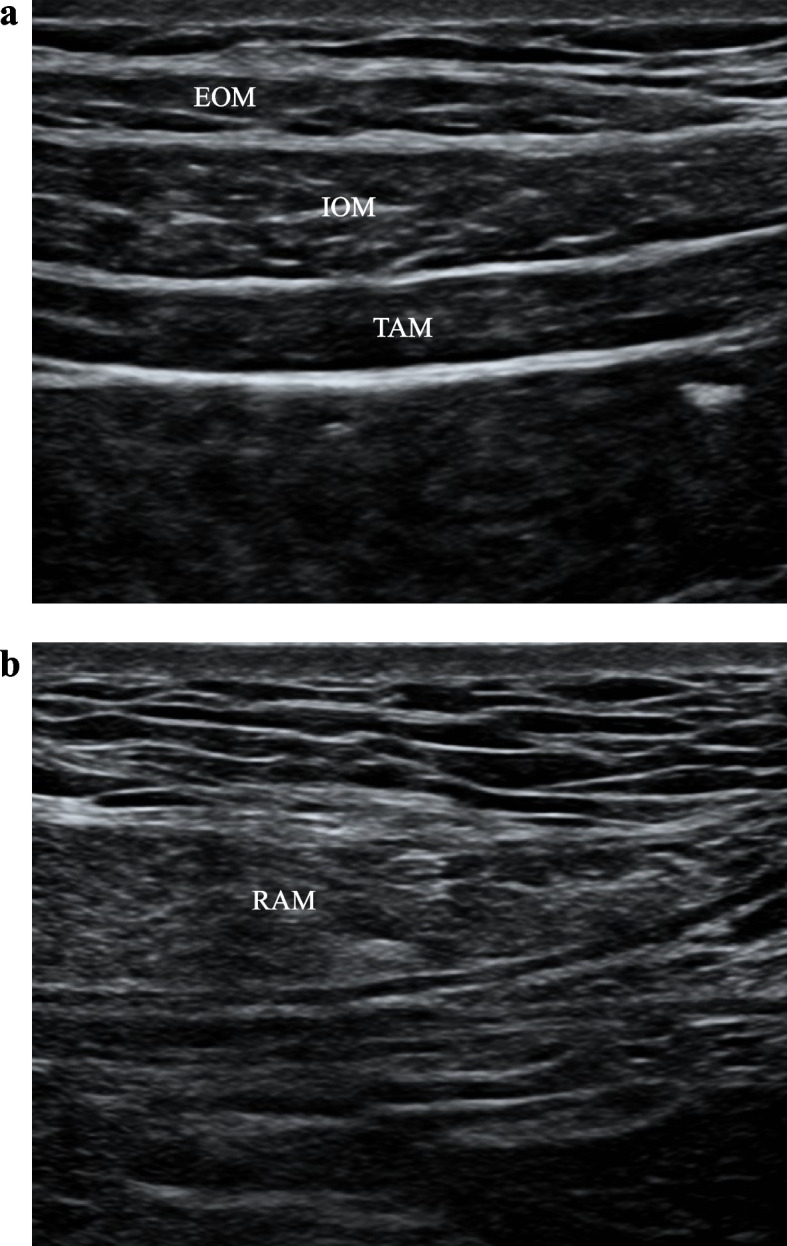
a Ultrasonographic image taken before the transversus abdominis plane block (TAPB). b Ultrasonographic image taken before the rectus sheath block (RSB)
